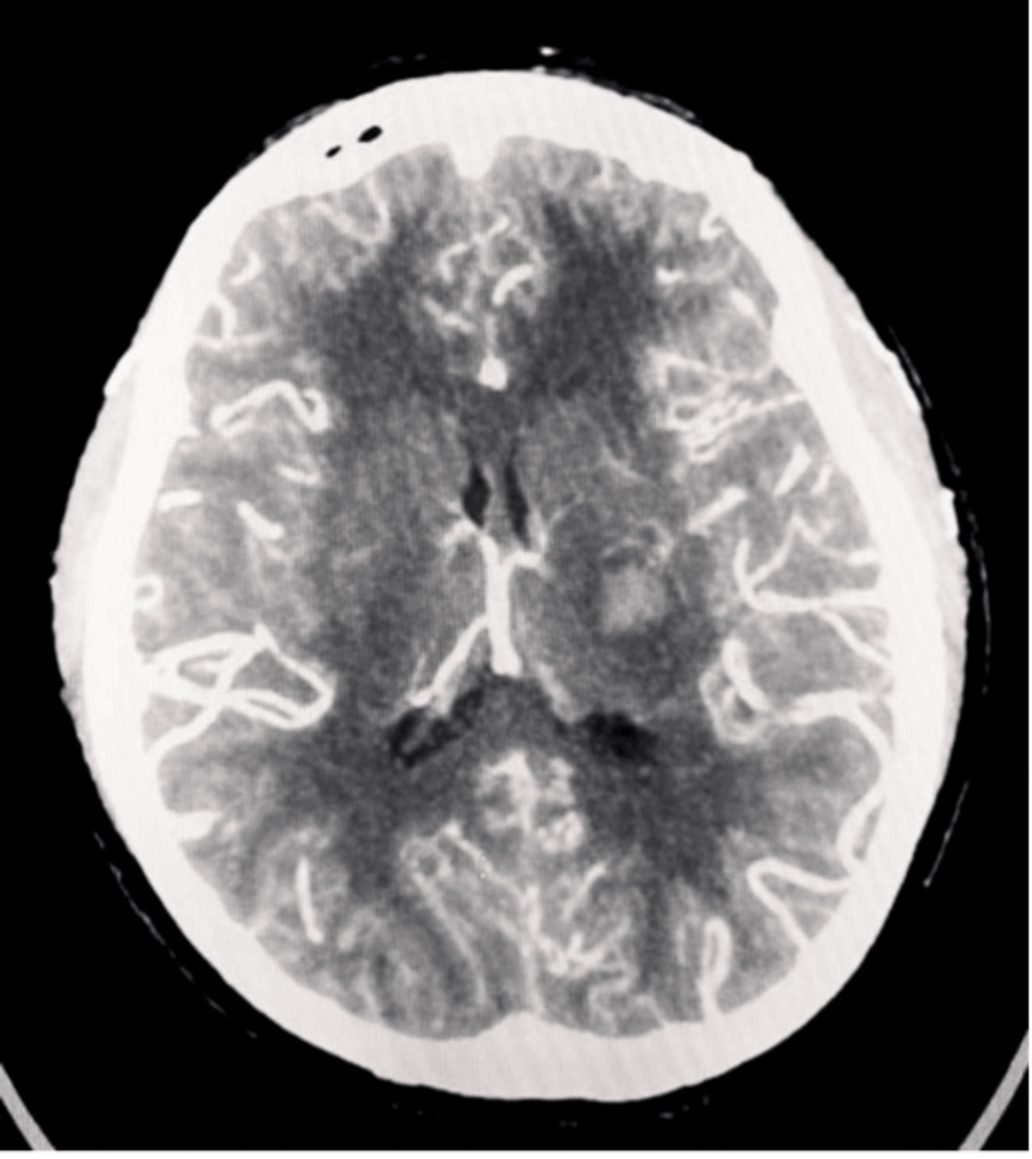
Axial CT angiography showing extensive collateral circulation with picture of 'puff of smoke'.
